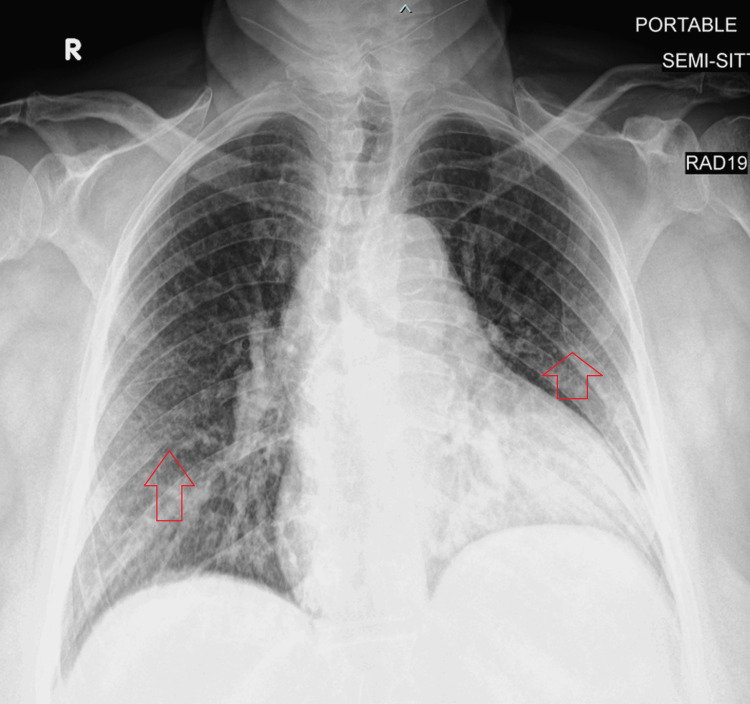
Portable anteroposterior chest X-ray showing bilateral interstitial changes with no consolidation
